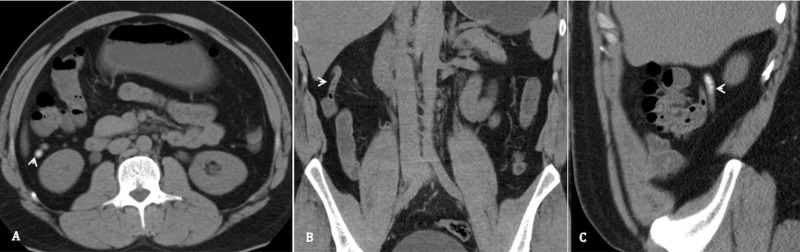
Incidental appendicolith Unenhanced CT abdomen, axial section (A), coronal section (B) and sagittal section (C), of a 23-year-old female with left lumbar pain. It is demonstrating a non-inflamed appendix (arrow head) with hyperattenuating tiny appendicoliths in its lumen.
